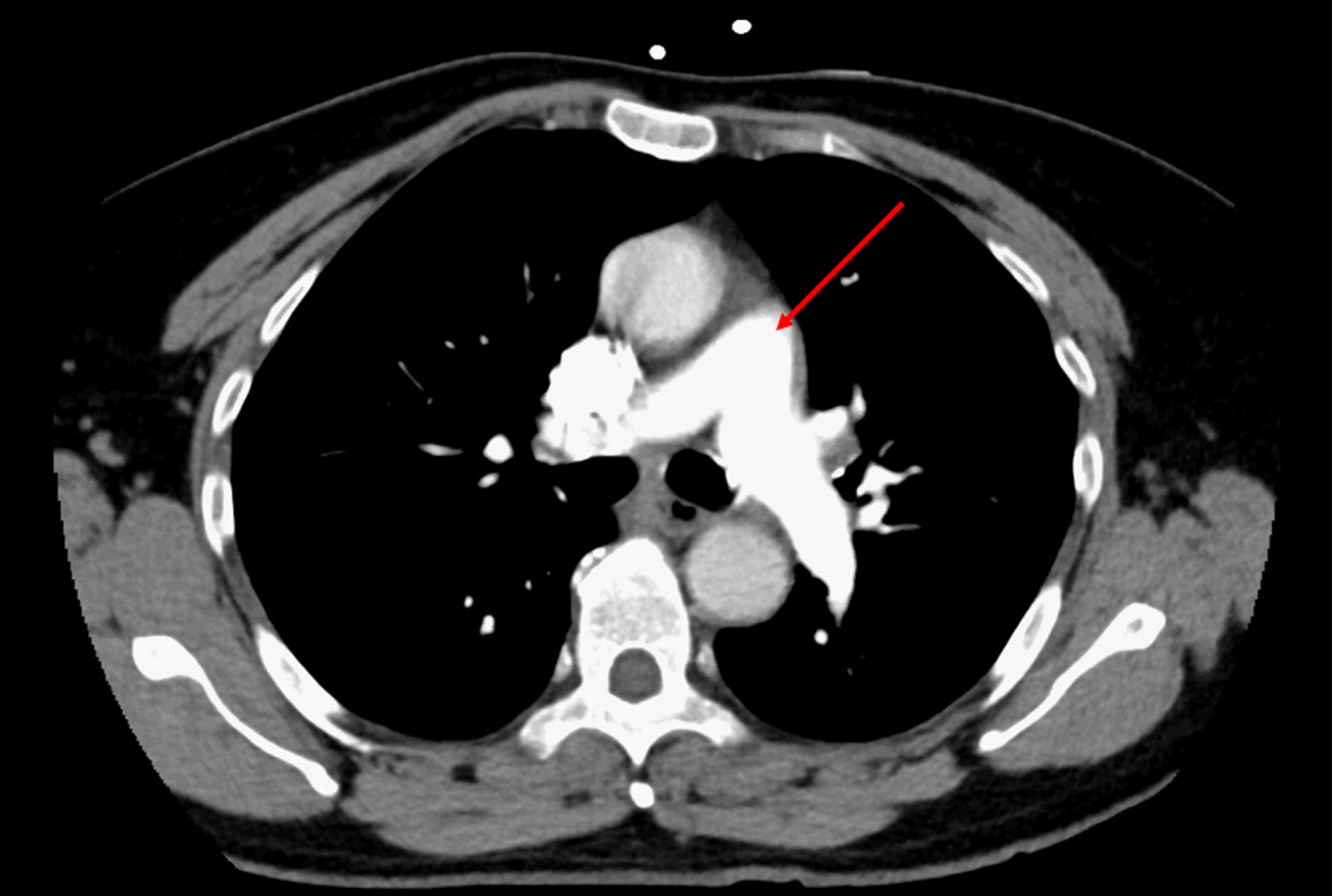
CT scan with pulmonary embolism protocol The arrow marks main pulmonary artery dividing into right and left branches. Lack of filling defect indicates the absence of pulmonary embolism.
